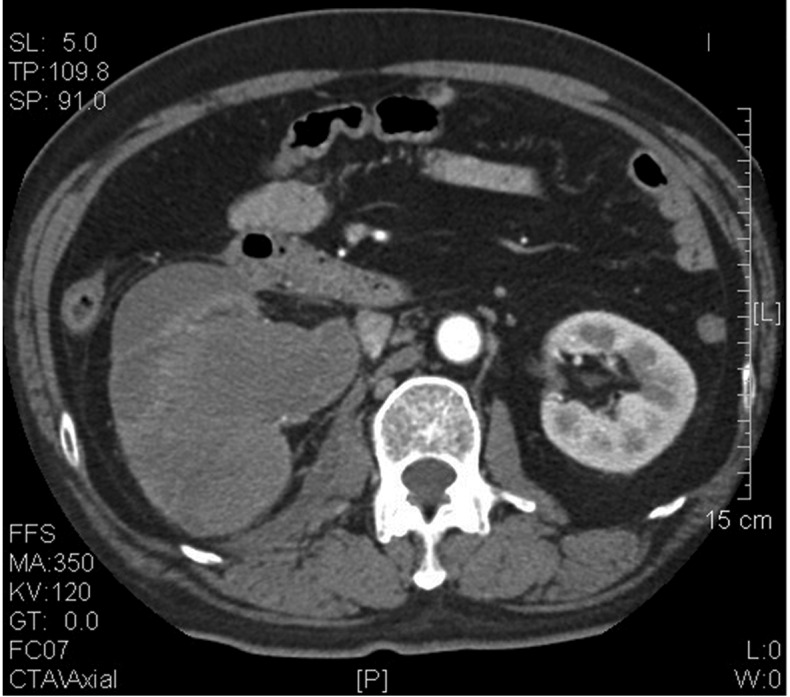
Pre-operative computed tomography examination of patient 1, showing a mass in the right renal pelvis, indicating a right renal pelvic carcinoma.
